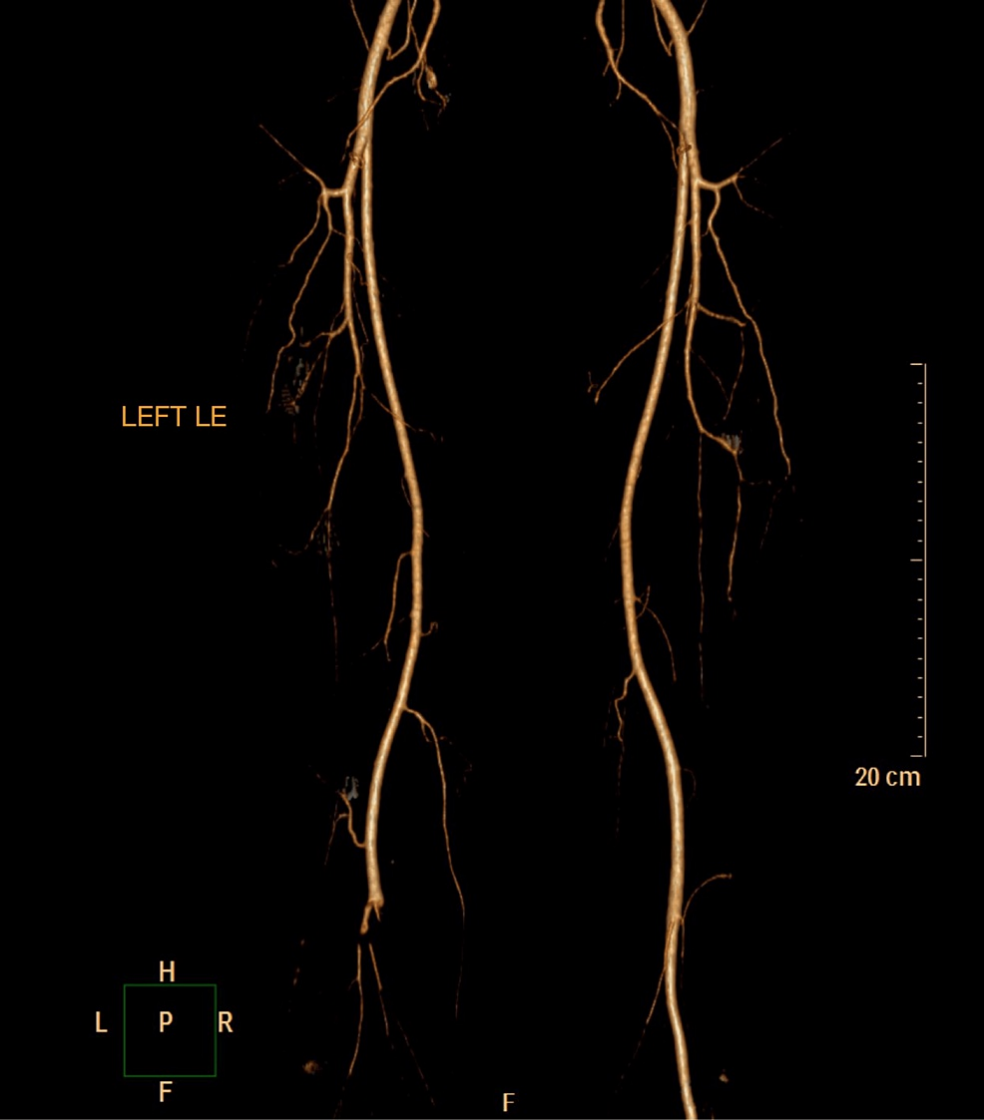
Computed tomography angiography (CTA) revealing left popliteal artery entrapment
